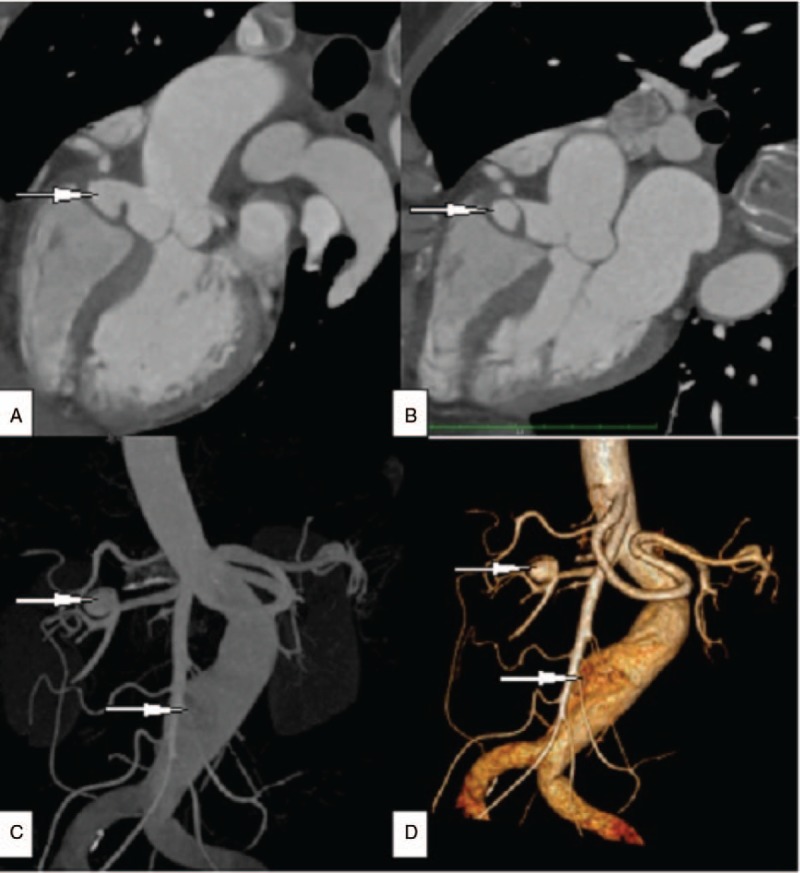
(A, B) CTA showed an aneurysm in the opening of RCA and leads to a severe stenosis of the ostium of the right coronary. (C, D) An aneurysm in the distal segment of right renal artery and an aneurysmal dilatation in the third lumbar plane of abdominal aorta in the CTA. CTA = computer tomography angiography RCA = right coronary artery.
